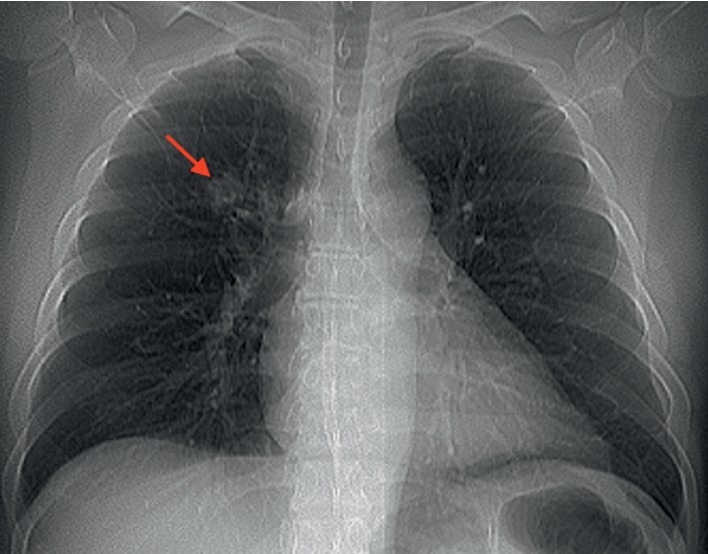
Chest X-ray showing a new right upper lobe nodule (red arrow).
